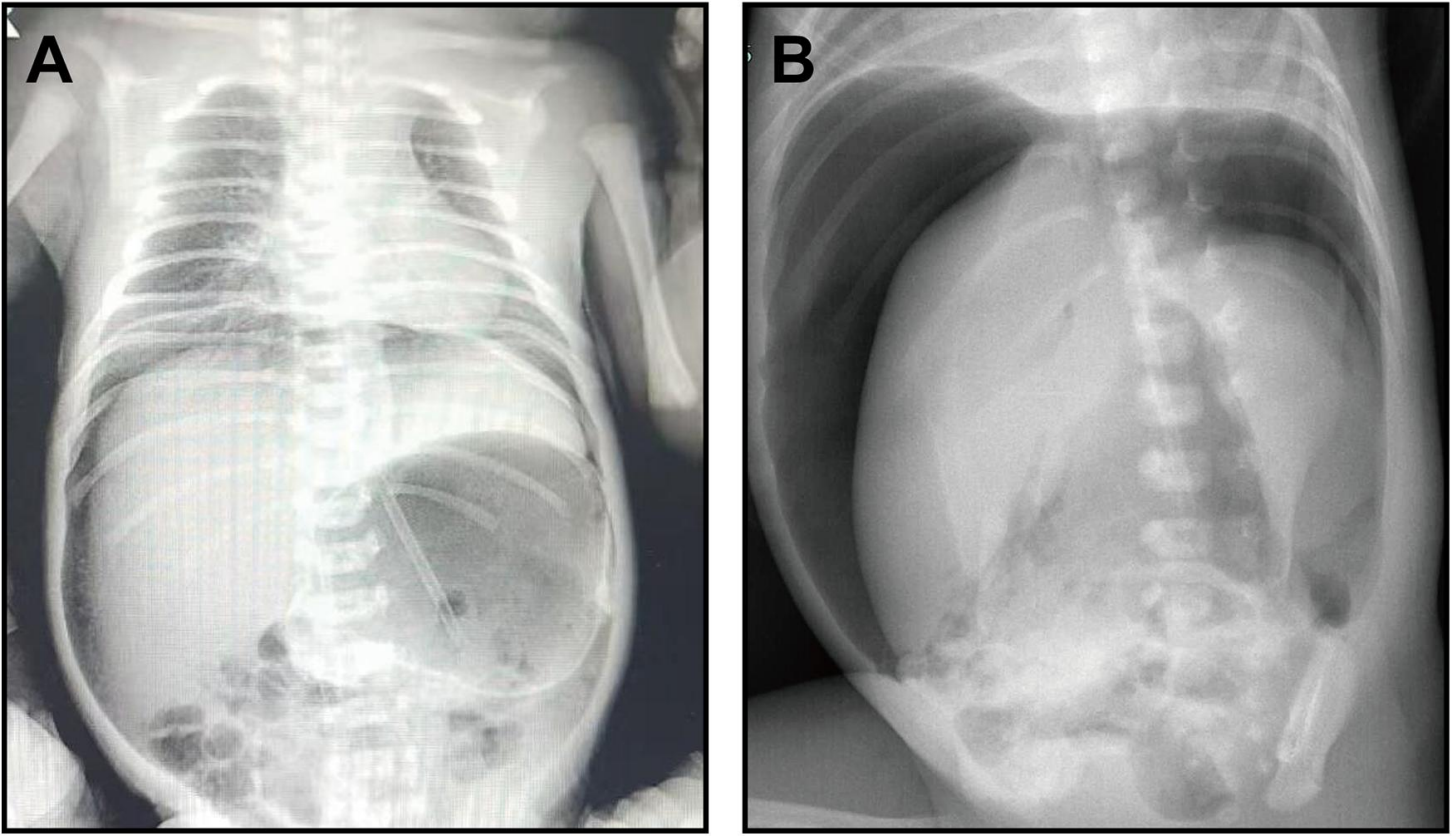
Preoperative abdominal radiographs demonstrating pneumoperitoneum. (A) An initial abdominal radiograph obtained at a referring hospital, revealing pneumoperitoneum. (B) A follow-up radiograph upon admission to our center, showing massive subdiaphragmatic free air and the classic “football sign”, indicative of spontaneous neonatal gastric perforation (SNGP).
